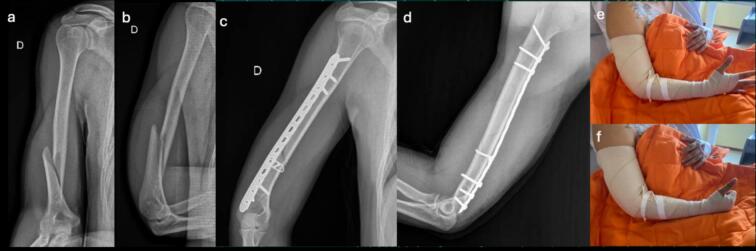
Case 2 – Male patient, 31 years old, mechanism of injury: arm wrestling. a-b) Preoperative AP and lateral radiographs showing AO 1.2.A.1-c fracture; c-d) Immediate postoperative AP and lateral radiographs; e-f) Immediate postoperative evaluation of radial nerve motor function.
